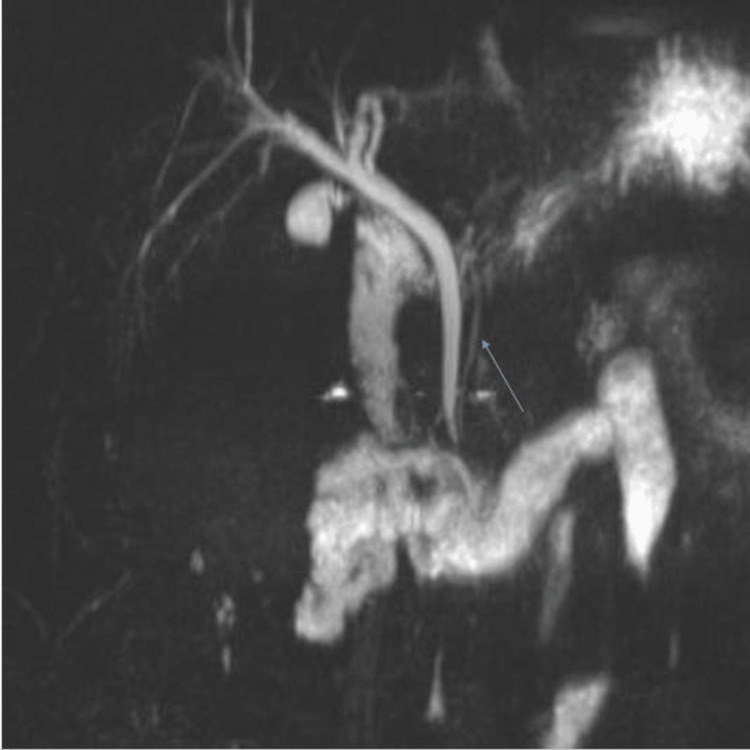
Coronal heavily T2-weighted MR cholangiopancreatography (MRCP). Image demonstrating a low insertion of the common bile duct, draining into the third portion of the duodenum with the pancreatic duct (arrow) seen to converge with the common bile duct upstream of the ectopic orifice.
